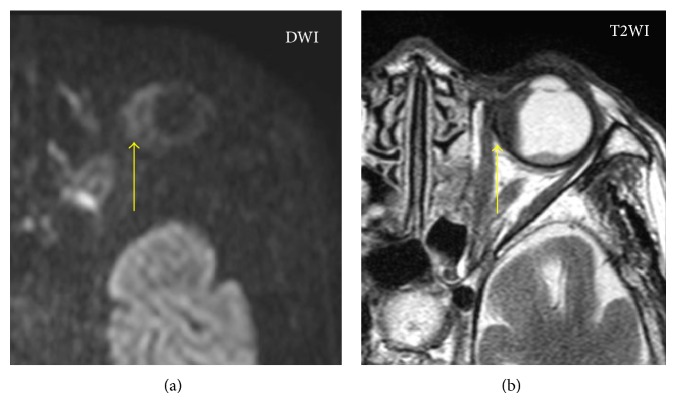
MRI images of the choroidal mass. Diffuse-weighted images (DWI) showed high signal intensity in left interior orbital lesion. T2-weighted image (T2WI) showed the choroidal tumor with low intensity of left eye.
